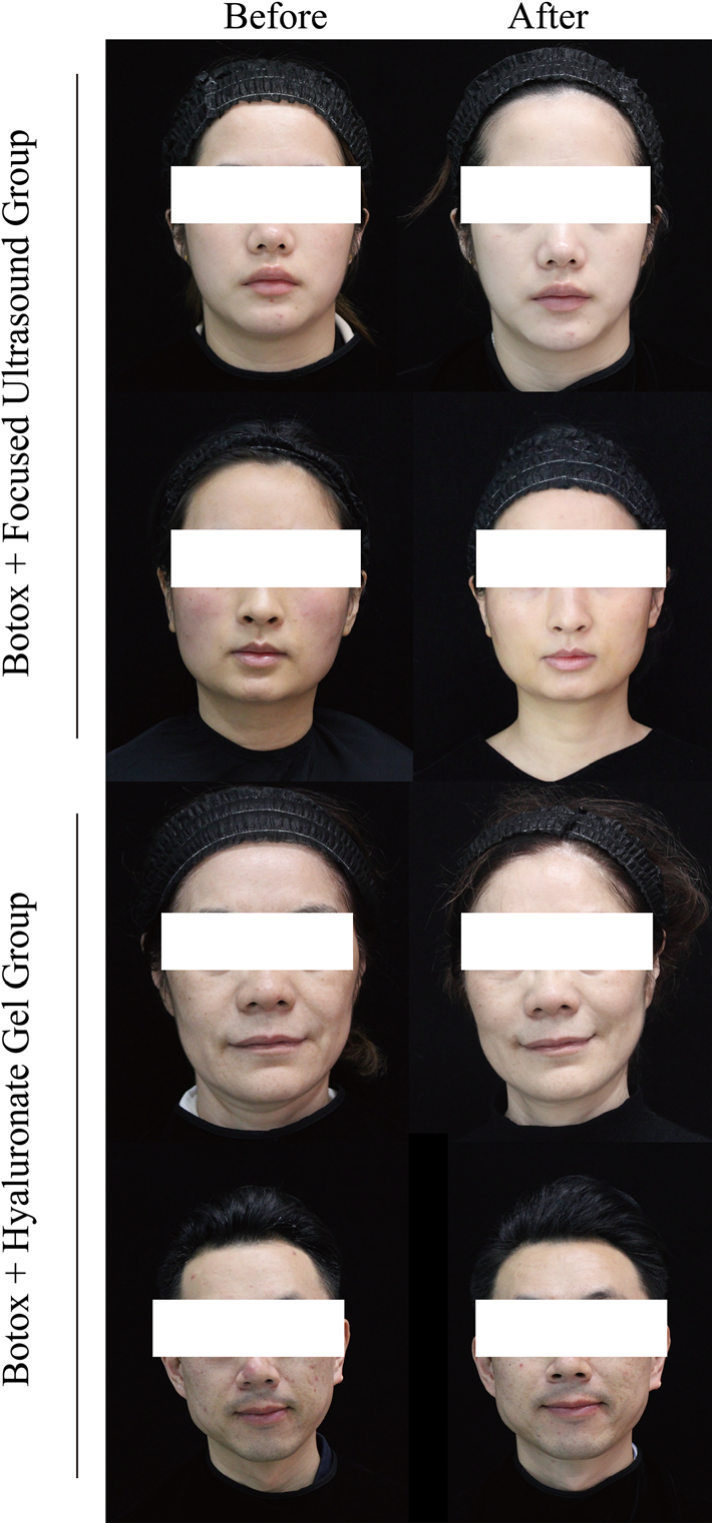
Pre- and post-treatment images of representative patients, including botox + focused ultrasound group and botox + hyaluronate gel group
